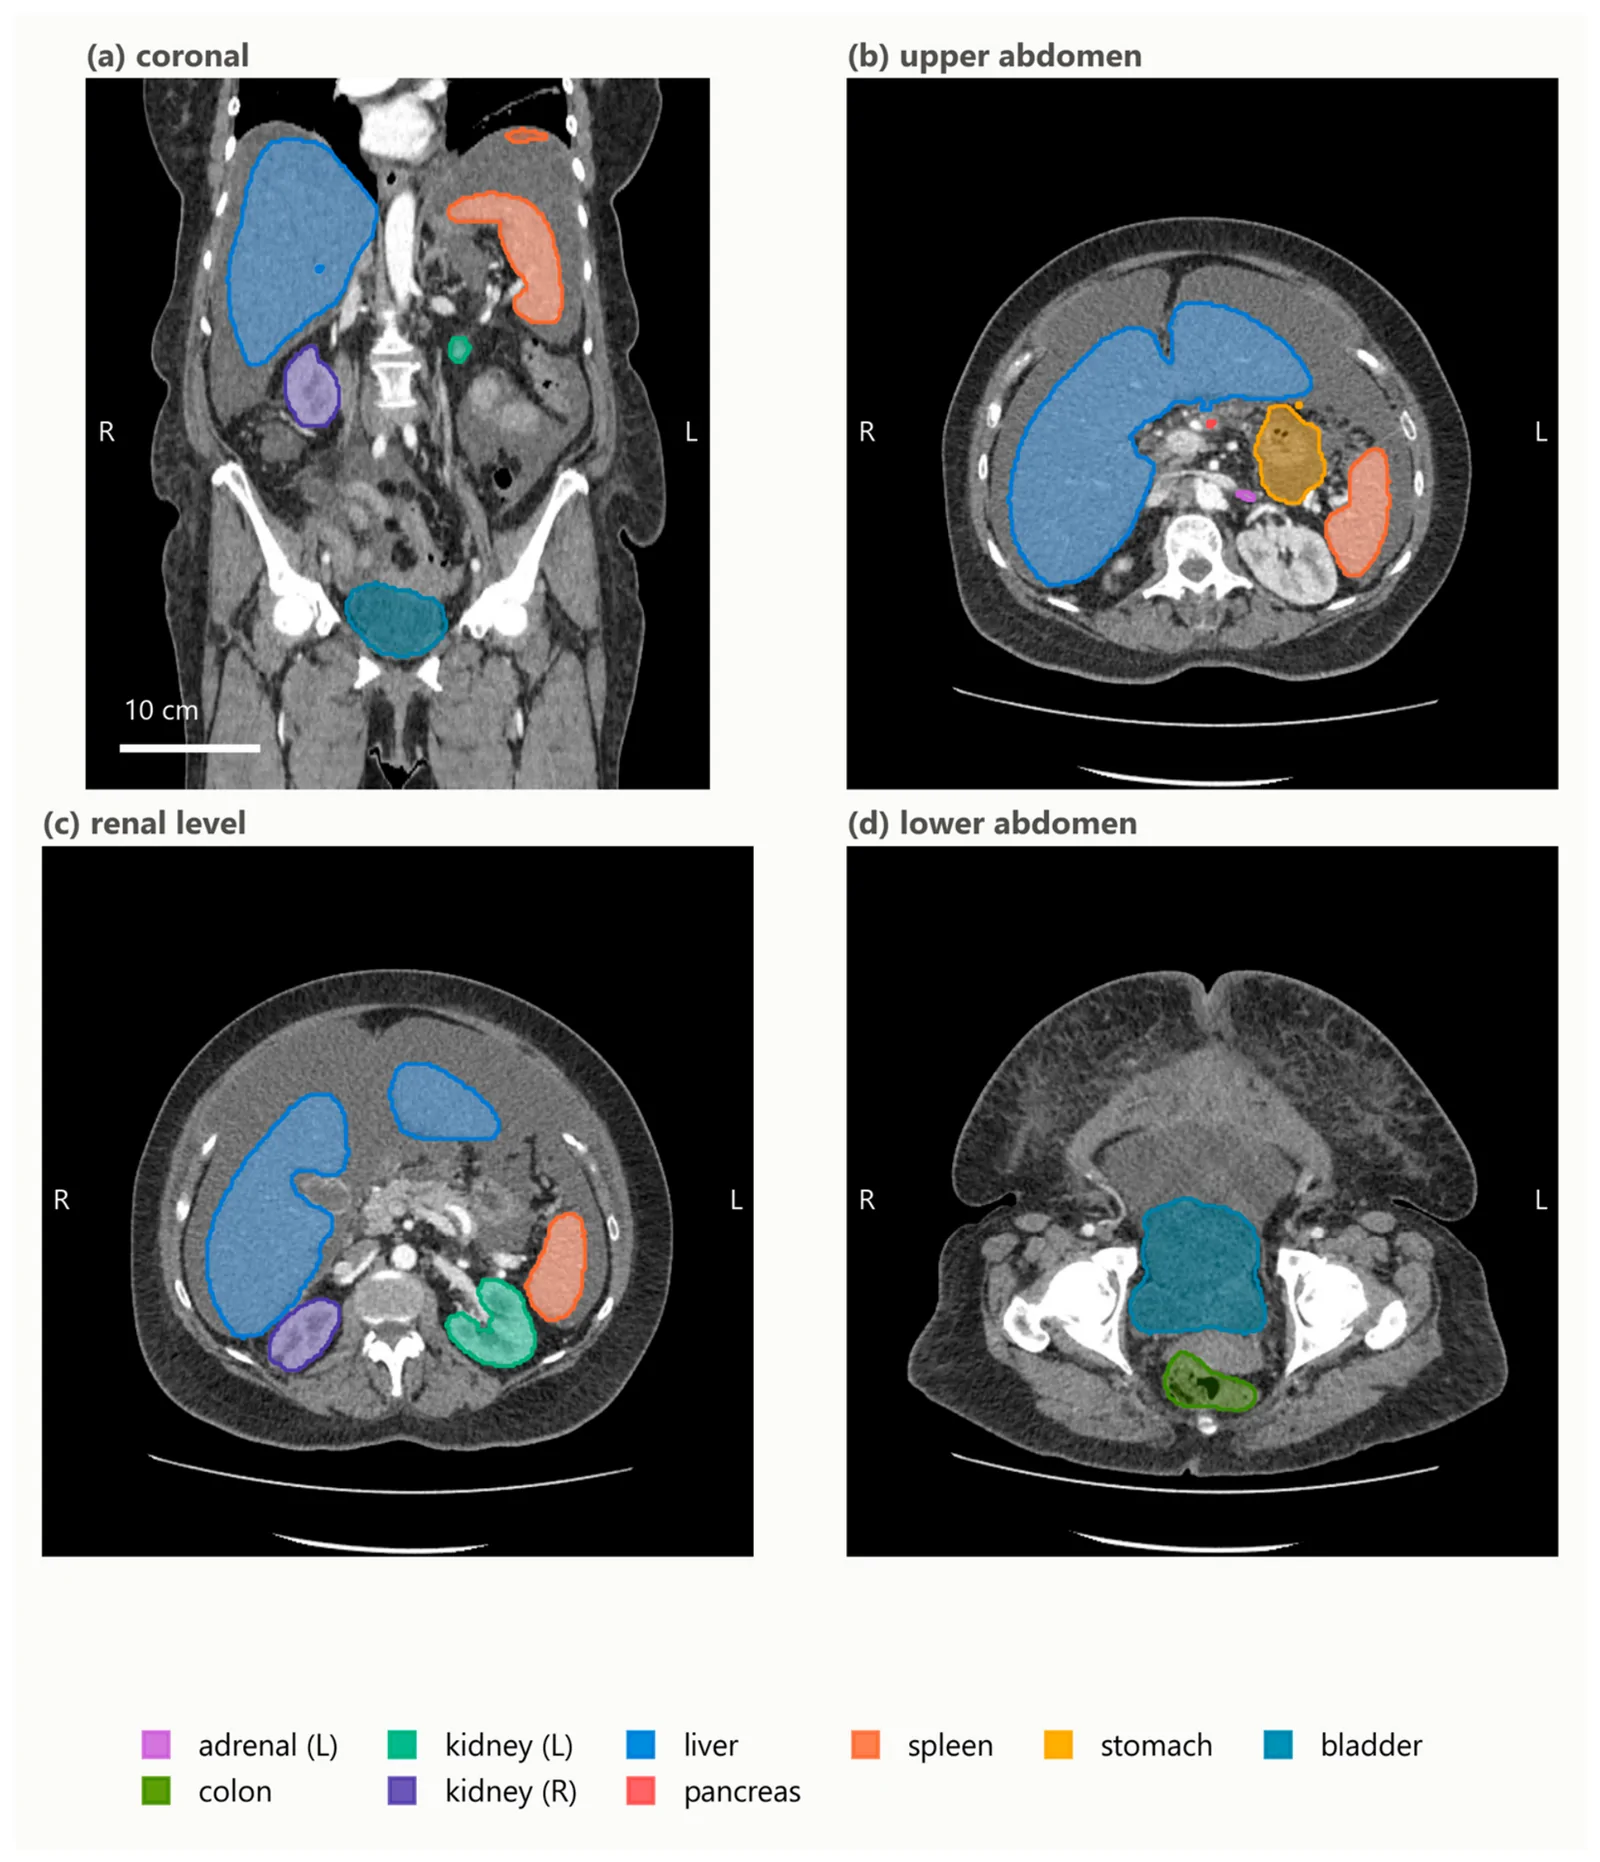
The representative TotalSegmentator output from one abdominal CT series used in the analysis. (a) The coronal reformat with multi-organ overlays. (b–d) Axial levels through the upper abdomen, the renal level, and the lower abdomen. Masks were generated with TotalSegmentator v2.17 using the 1.5 mm full-resolution total task and returned to the native DICOM-derived image grid; overlays are translucent so that the anatomy they are drawn against remains visible and the key lists of the structures actually shown. The same acquisition appears in Figure 3. Images are displayed in radiological convention, with the patient’s left on the viewer’s right. Only de-identified imaging from the Cancer Imaging Archive is shown.
